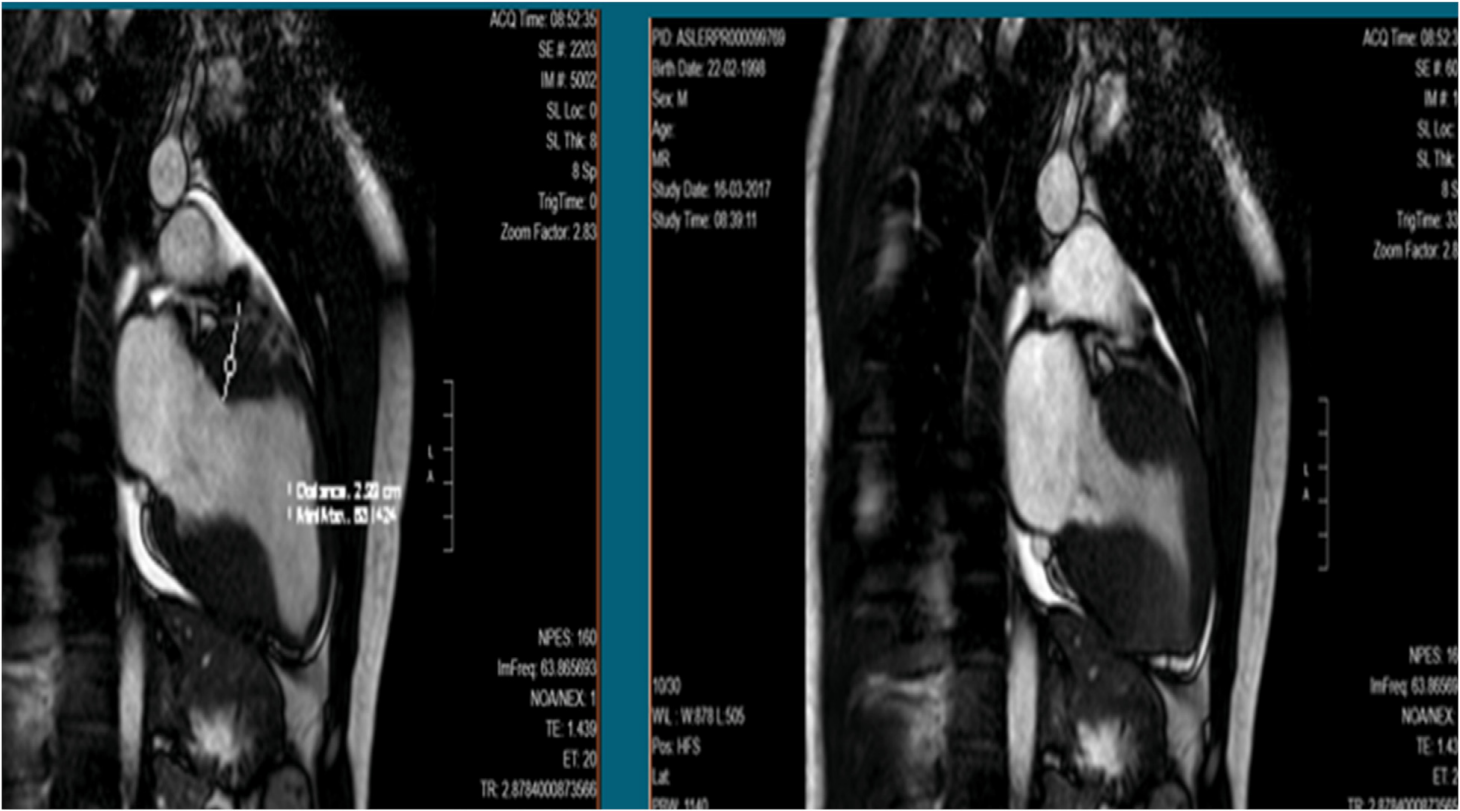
MRI reveals an evolving condition with an increased mid-basal parietal thickness up to 23–25 mm compared to the previous exam, with fibrosis in the inferior septal wall.
